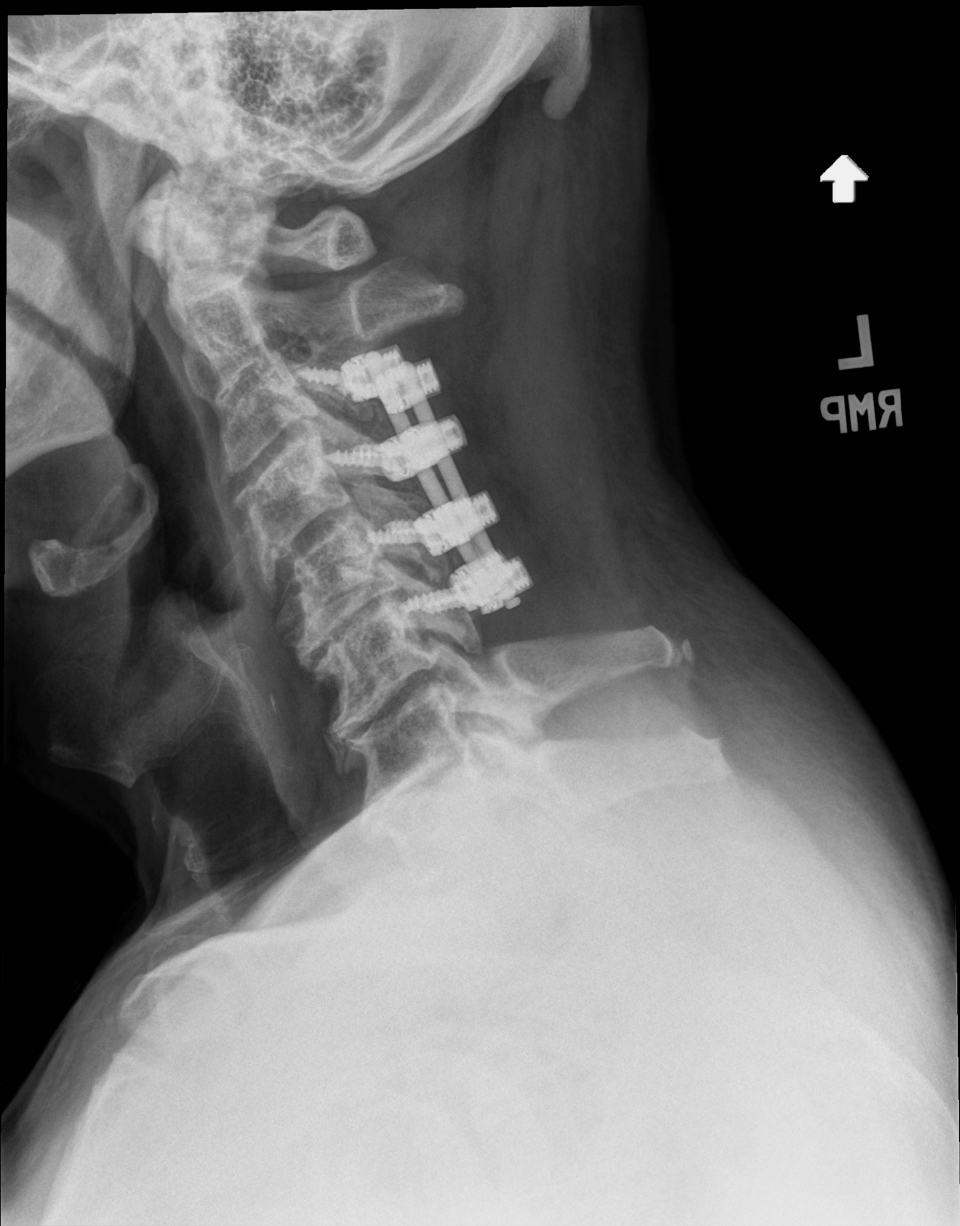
Postoperative radiography showing posterior spinal decompression and fusion spanning C3-C6.
